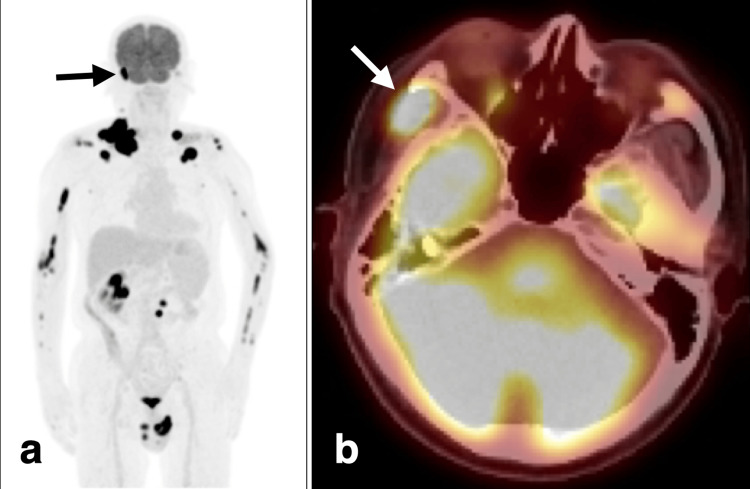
FDG-PET/CT two months after CKR (a) MIP. Multiple lesions with high FDG uptake in the whole body MIP. There was high FDG uptake of the lesion in the right temporal fossa on PET/CT (black arrow). (b) The right temporal lesion with high FDG in the PET/CT (white arrow). FDG: 2-deoxy-2-[18F] fluoro-D-glucose, PET: positron emission tomography, CT: computed tomography, CKR: CyberKnife radiotherapy, MIP: maximum intensity projection
